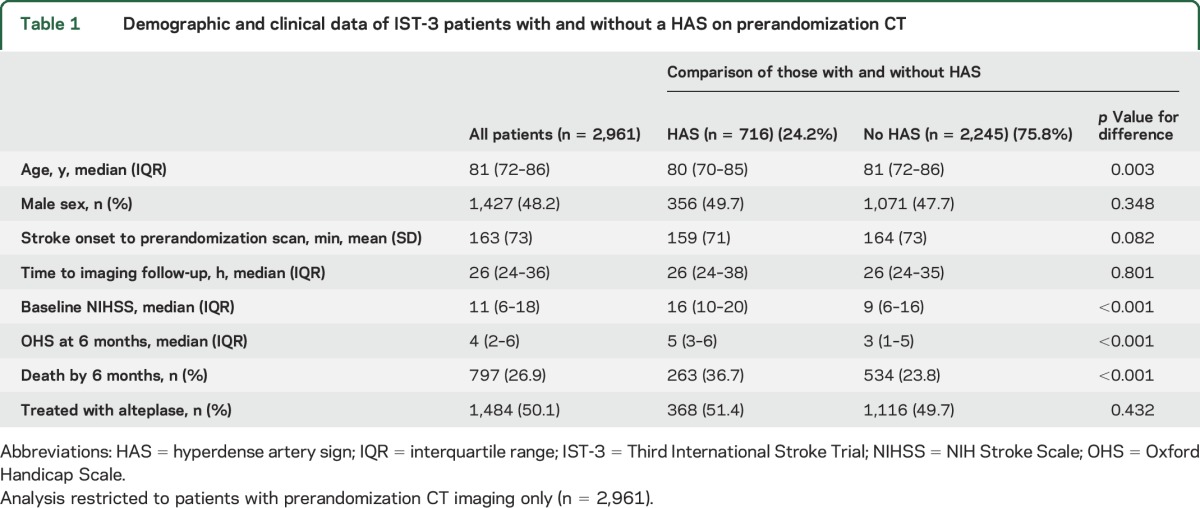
Demographic and clinical data of IST-3 patients with and without a HAS on prerandomization CT
